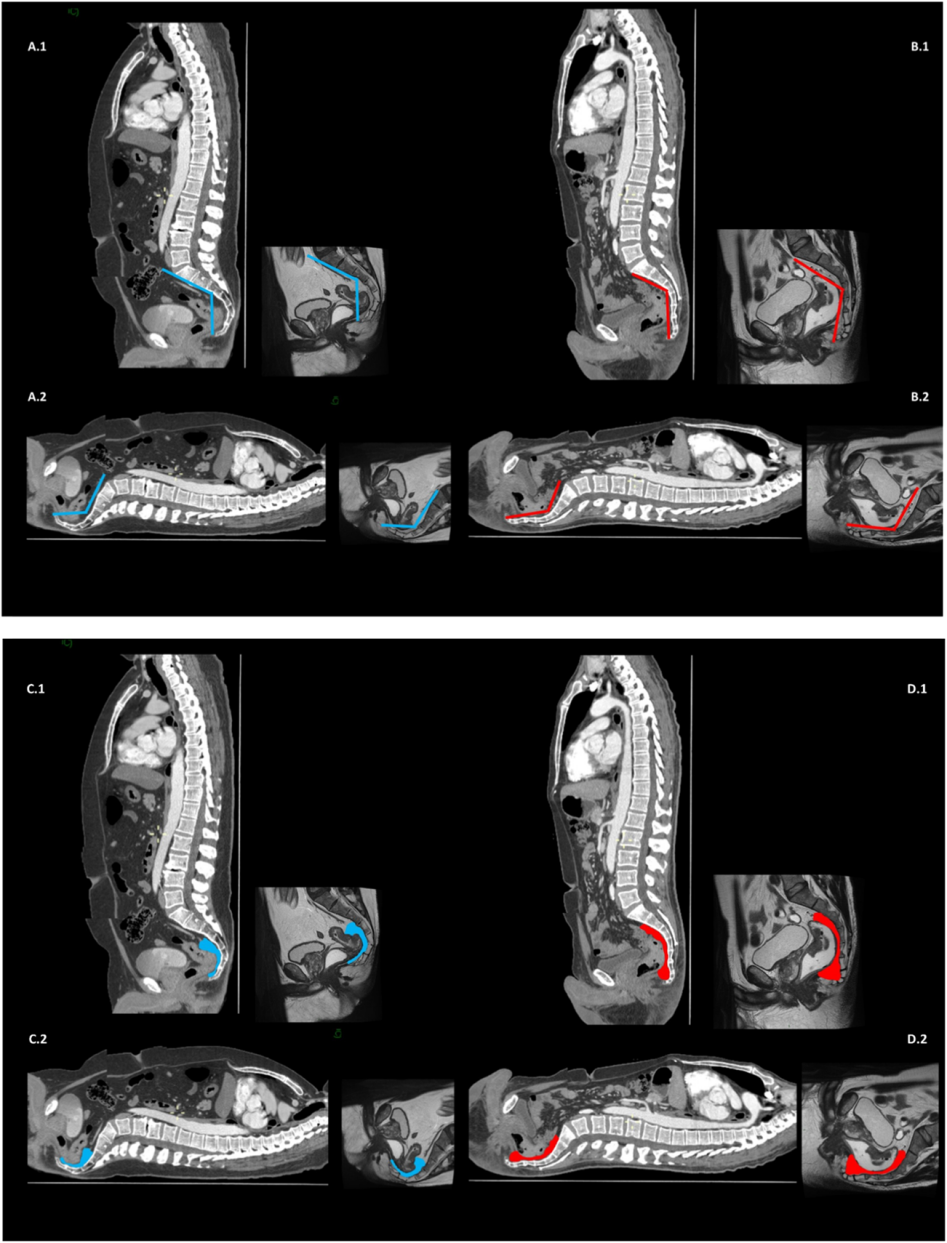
CT and MRI scans in a patient with rectal cancer documenting angle *ε* and posterior mesorectum. A1. Sagittal plane in a male patient with an acute angle *ε* represented by vectors on CT and MRI scans. A2. Same images showing difference when ideally approaching the rectum bottom-up. B1. Sagittal plane in a male patient with an obtuse angle *ε* represented by vectors on CT and MRI scans. B2. Same images showing difference when ideally approaching the rectum bottom-up. C1. Same patient presented in A1 and A2 with acute angle *ε*, highlighting posterior mesorectum, and C2 the difference when ideally approaching the rectum bottom-up. D1. Same patient presented in B1 and B2 with obtuse angle *ε*, highlighting posterior mesorectum, and D2 the difference when ideally approaching the rectum bottom-up
